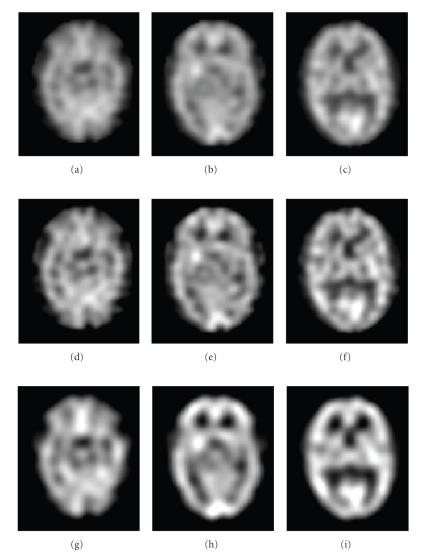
Restoration results. Axial view of: (a), (b) and (c): Original SPECT cross-sectional images. (d), (e) and (f): deconvolution result with Metz filter. (g), (h) and (i): deconvolution result with our method.
